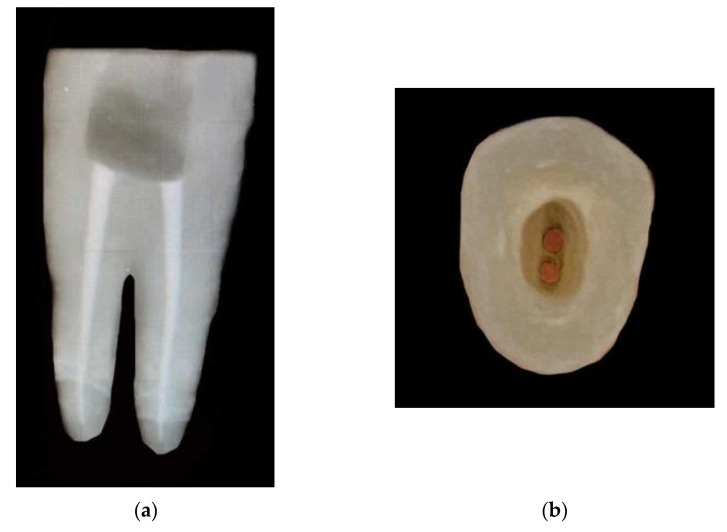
(a) Radiographic proximal view of Endocrown preparation without intraradicular extension. (b) Occlusal view of Endocrown preparation without intraradicular extension.
